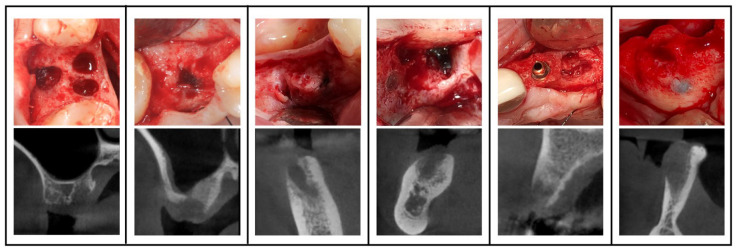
Clinical view of 5 cases with incomplete healing with black, tar-like substance in the socket and corresponding CBCT cross-sectional image (see pictures below) after the use of Terra-Cortril.
